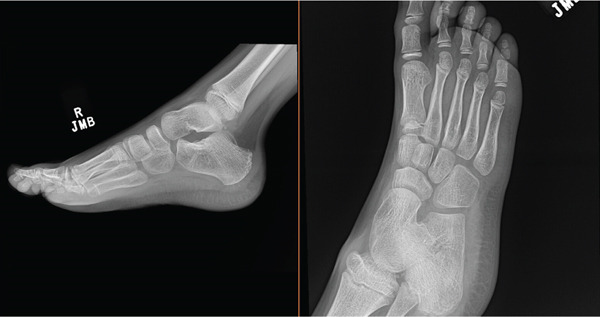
Lateral and oblique radiographs demonstrating lack of lesion recurrence and preservation of joint spaces at 1.5 years postoperatively.
